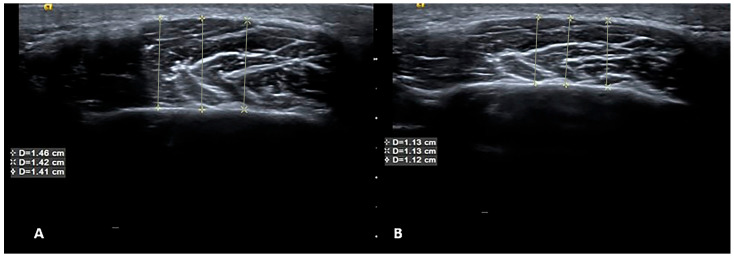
USG at baseline and 4 weeks after BT-A therapy. Ultrasonographic images of the masseter muscle before (A) and after (B) botulinum toxin injection. As seen from measurements listed in the text boxes superimposed on the images, muscle volume decreased after treatment, which indicates that the treatment was successful. Yellow Lines indicates the measurement of the muscles from different region.
